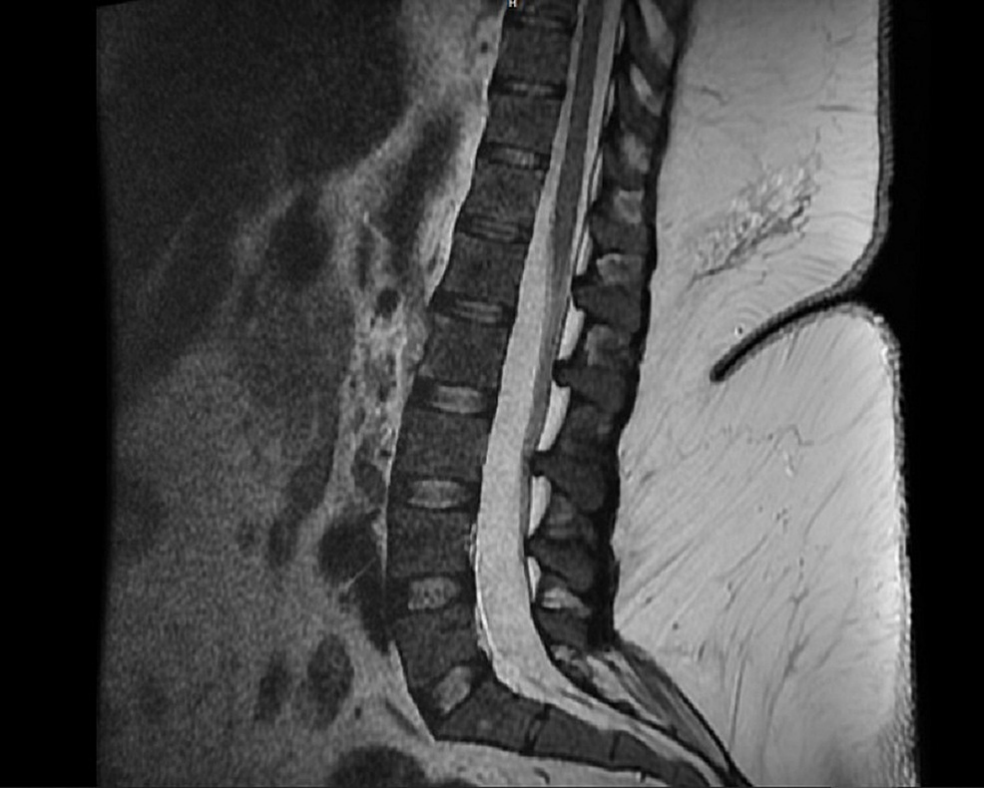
MRI Lumbosacral Spine MRI lumbosacral spine showing normal intervertebral disc spaces, vertebral bodies, and spinal cord.
